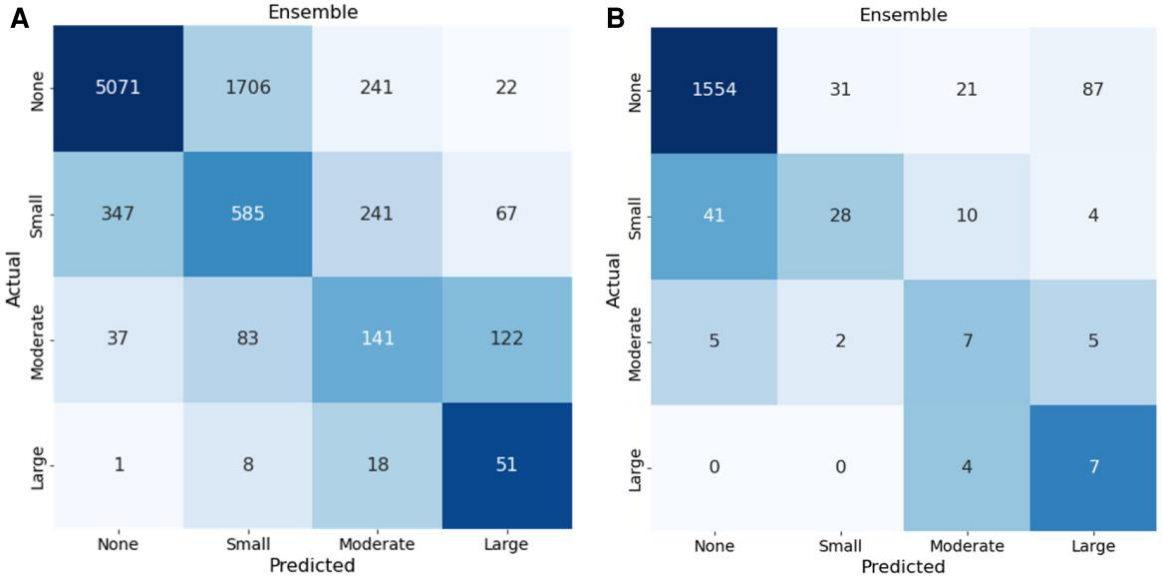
Confusion matrices for pericardial effusion severity classification in the primary cohort (*A*) and the external validation cohort (*B*). The EchoNet-Pericardium model utilizes an ensemble of predictions from five standard echocardiographic views to classify pericardial effusion as none, small, moderate, or large. *Figure 1A* demonstrates performance in the primary test cohort, while *Figure 1B* depicts results in the external validation cohort. The diagonal values indicate correct predictions, with the model showing consistent accuracy across different levels of effusion severity.
